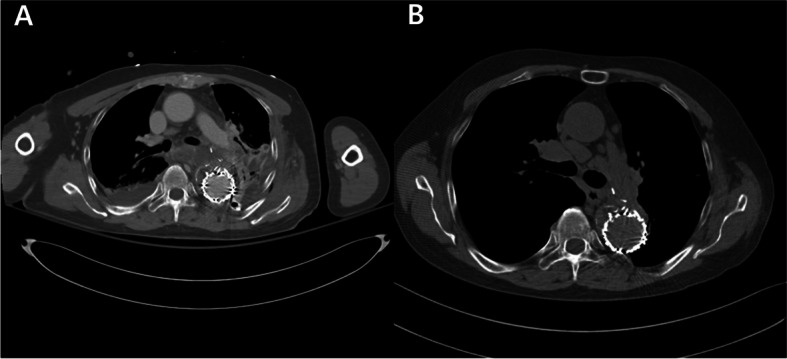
Postoperative CT findings. a Complete absence of any remaining endoleak and a well-repaired descending thoracic aorta before discharge. b Non-enhanced CT imaging at 6-month outpatient follow-up showing no size changes in the descending aorta. CT: computed tomography
